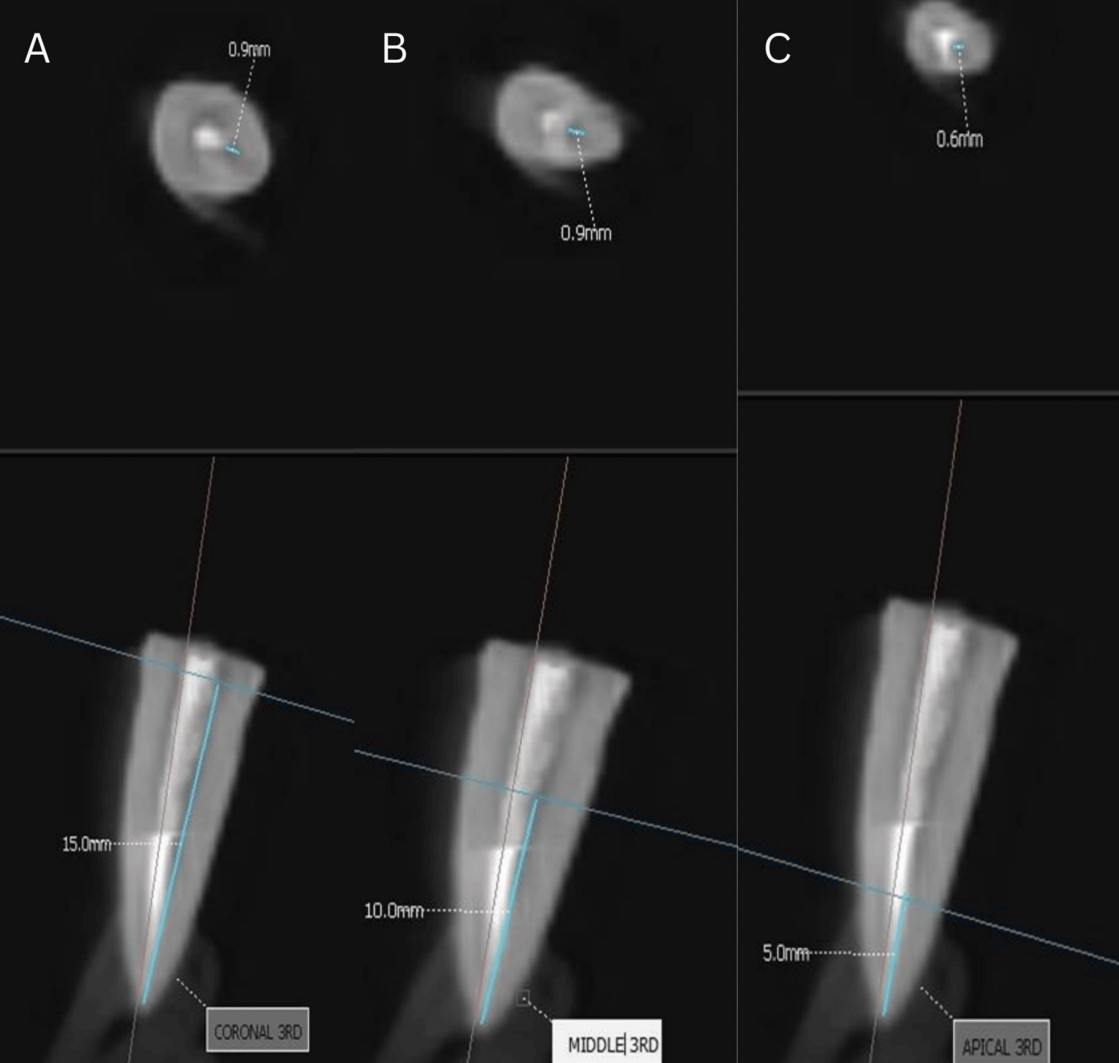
CBCT analysis of warm vertical compaction technique CBCT images showing obturation with the warm vertical compaction technique in the (A) coronal third, (B) middle third, and (C) apical third.
